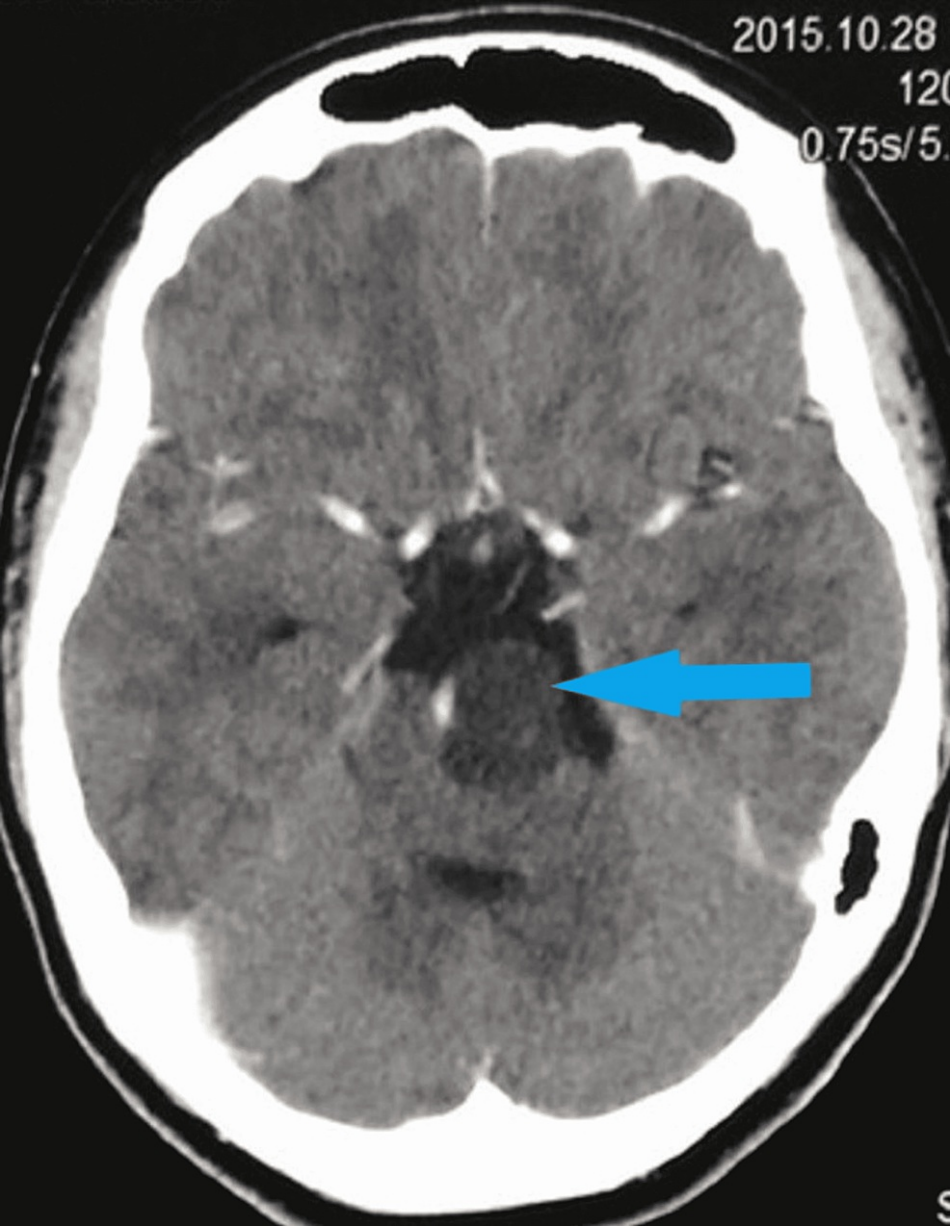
Contrast CT scan of the brain shows a non-enhancing hypodense lesion in the pre-pontine cistern. CT: computed tomography
